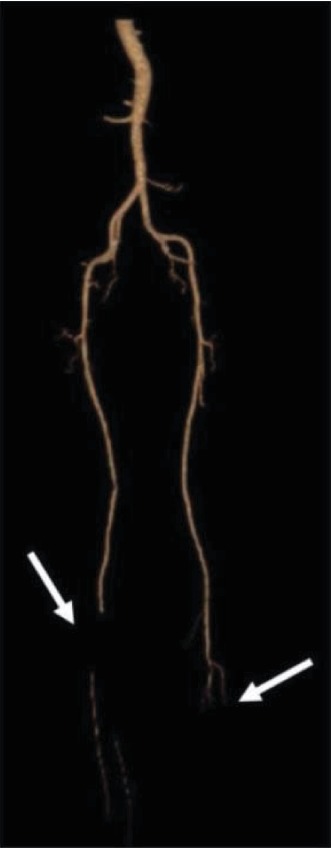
Computed tomography angiogram of the lower extremities showing a perfusion defect in the right tibial artery and left popliteal artery.
